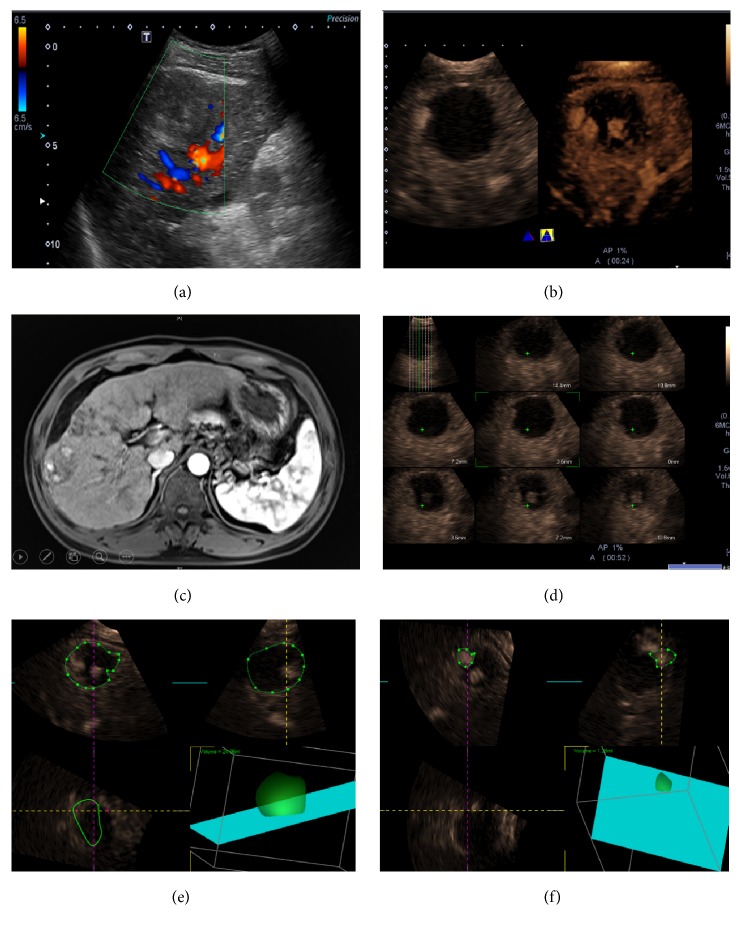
Residual tumor after radiofrequency ablation (RFA). Conventional ultrasound image in a 65-year-old man showed a 45 mm sized homogeneous hypoechoic lesion in the right hepatic lobe. No color signal can be detected inside the lesion by color Doppler flow imaging (CDFI) (a). There was a small enhanced area along the border of the lesion on dynamic 3D-CEUS (right) other than 2D-CEUS (left) images (b). Contrast MR imaging showed confirmed RT inside the lesion with local enhancement (c). The “multislice” display mode showed the RT area on several slices of 3D-CEUS image (d). The contour of the whole lesion was depicted manually and its corresponding volume was calculated automatically (e). The volume of the 3 nodular RT area can also be calculated. The residual proportion added up to 13.4% (f).
